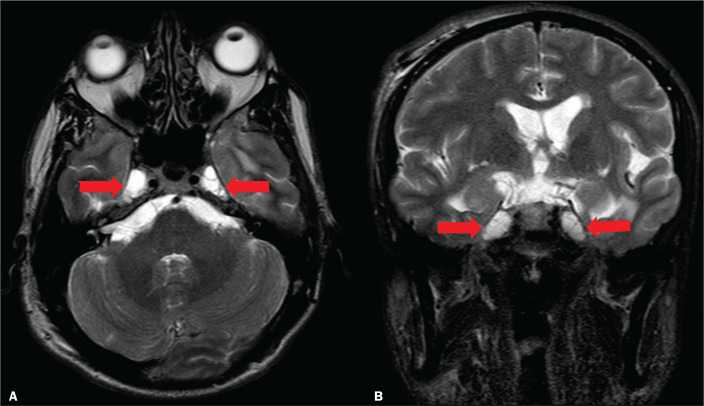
Axial and coronal T2-weighted MRI scans (A and B, respectively) showing
